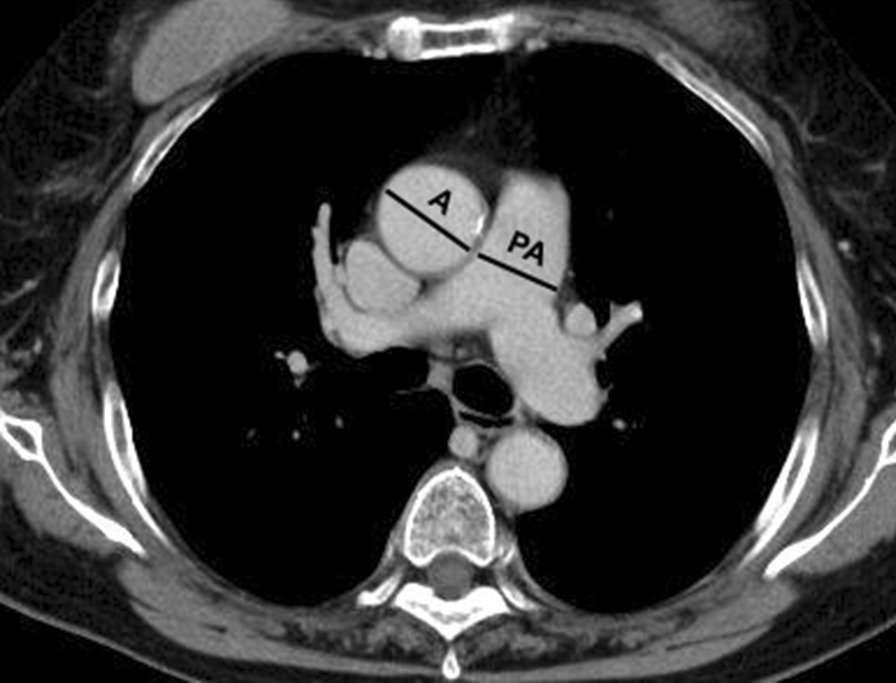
Axial chest CT image at the level of the left and right main pulmonary arteries. Measurements of the diameter of the main pulmonary artery (PA) and the diameter of the aorta (A) at the level of the bifurcation are used to calculate the PA:A ratio in accordance with a previous report [19]. If the diameter of A is not uniform, two measurements are taken 90 degrees apart, and the larger diameter is used
